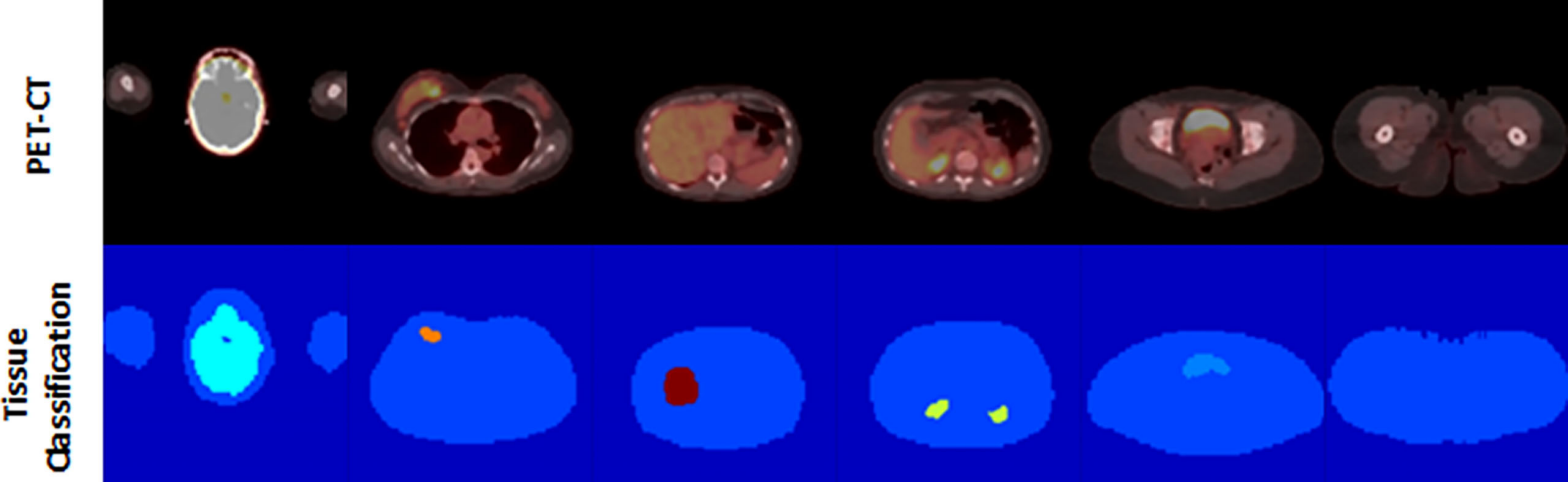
Example of PET/CT and tissue classification map pairings.
